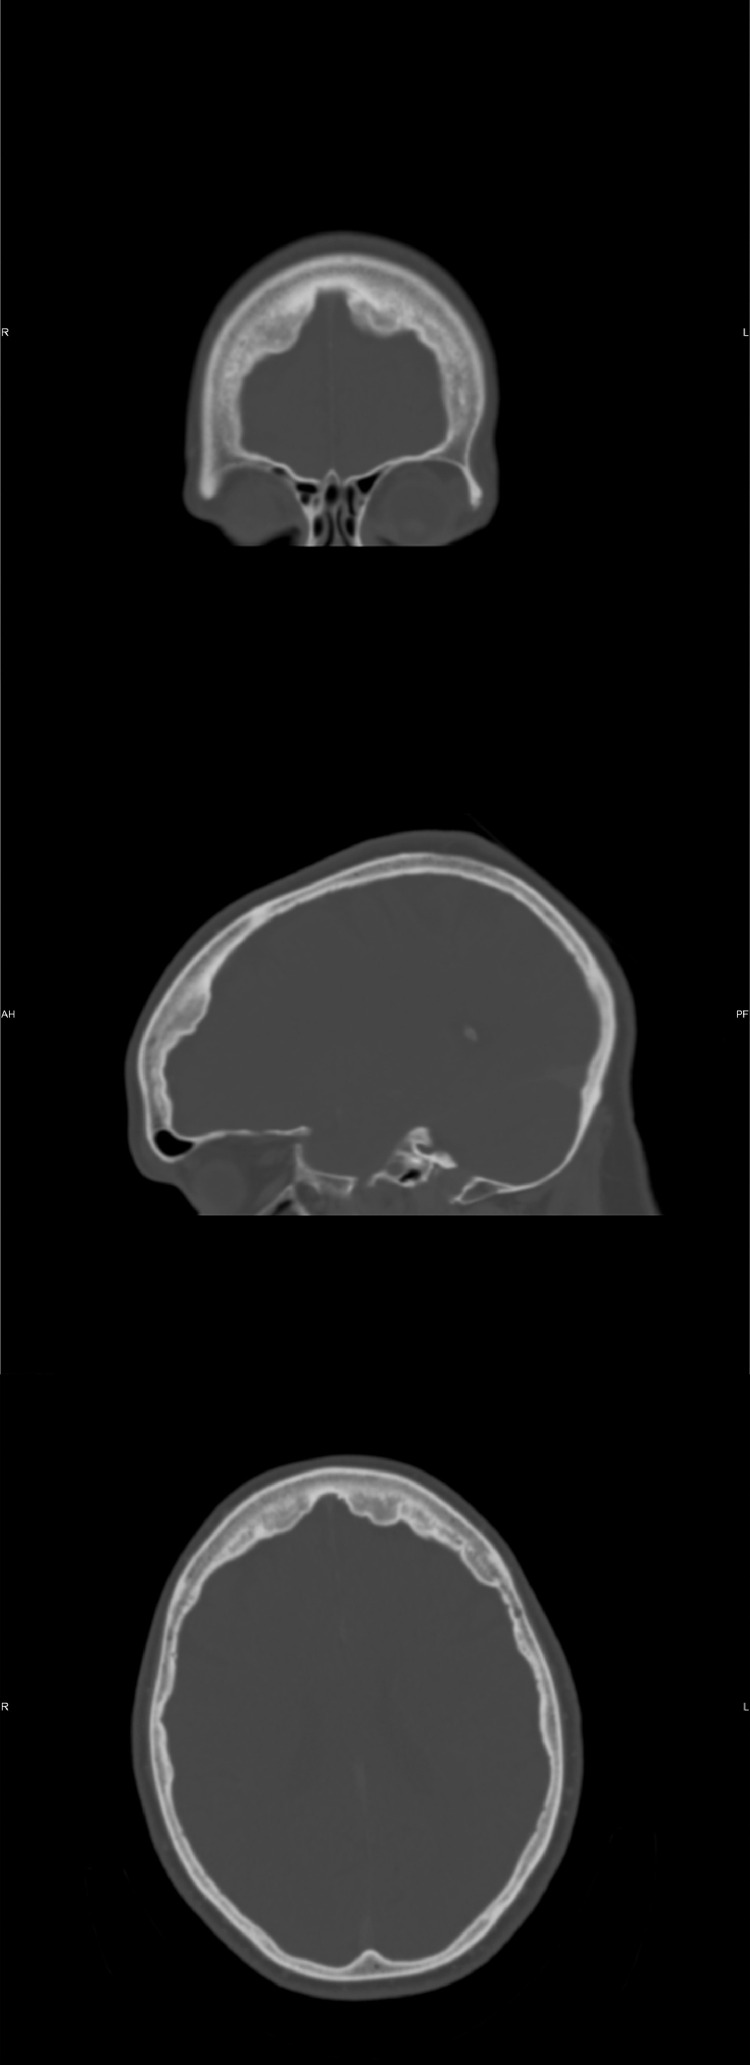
Sagittal, axial and coronal CT images of the skull show irregular, nodular thickening of the frontal bone inner table extending to involve the parietal bones, with sparing of the midline and outer calvarial surface.
